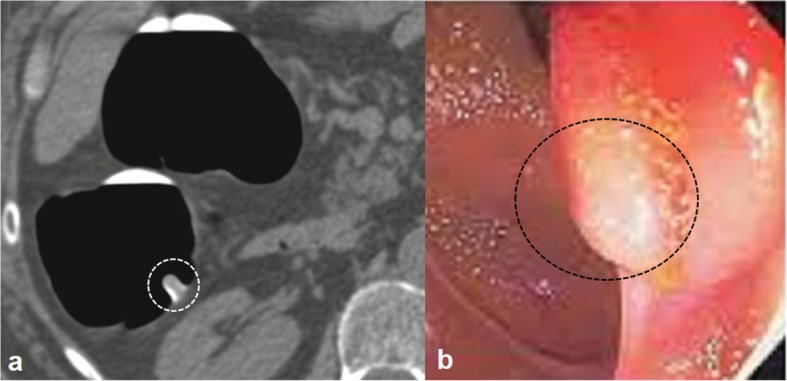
Colon lesion shouldering of 53 years old female. a: Computed tomographic colonography, the white circle indicates lesion shouldering. b. Colonoscopy, the black circle indicates lesion shouldering
